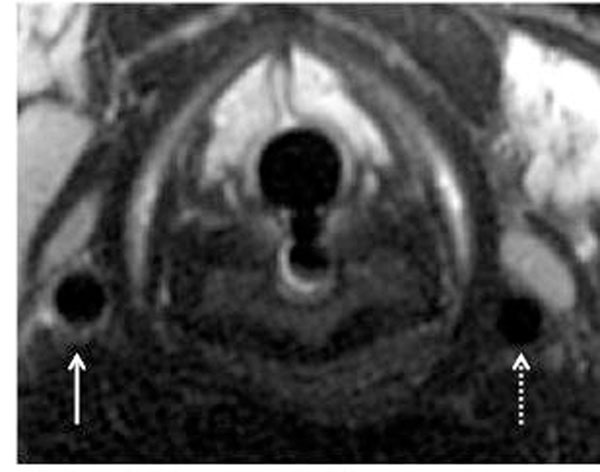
T2-STIR cross-sectional image of the carotid arteries from a single MRI examination four days post injury. Note that there is a large difference in SI between the injured (non-stippled arrow) and uninjured artery (stippled arrow).
